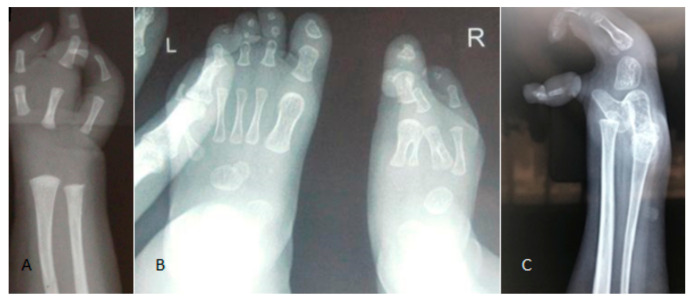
An AP hand radiograph of a six-month-old girl with GS showed a lobster claw hand with oligodactyly (aplasia of the second and fourth fingers with preservation of the thumb) and index and distal dysplasia of the fifth finger, associated with total aplasia of the carpal bones (A). An AP foot radiograph of a month-old girl showed a split foot deformity of the right foot (B). An AP foot radiograph of a 14-year-old girl with GS showed ectrodactyly (preservation of the big toe associated with tarsal and metatarsal agenesis) (C).
